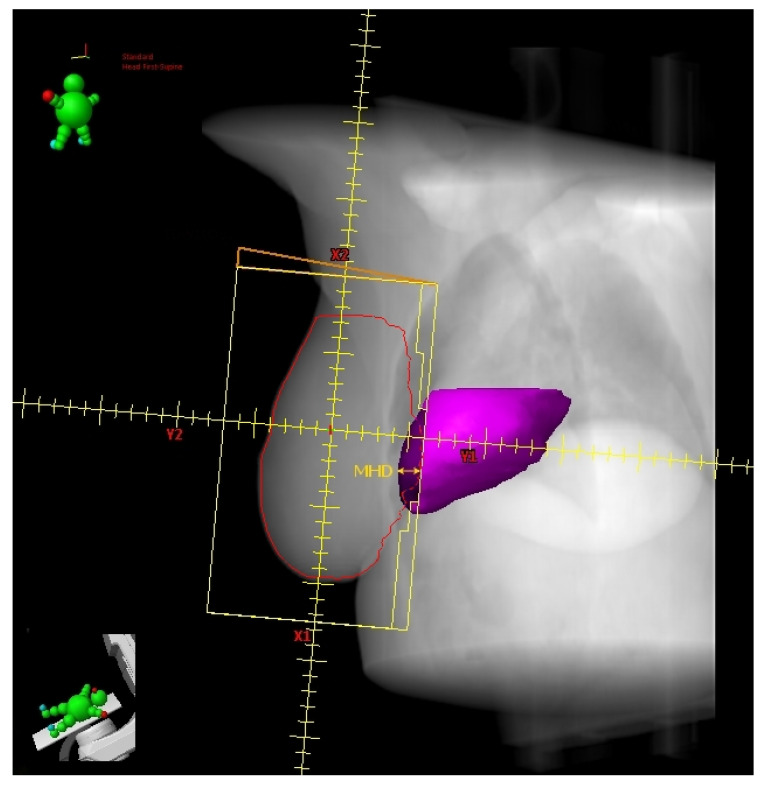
Digitally reconstructed radiograph (DRR) with external tangential beam’s-eye-view. The heart volume in field (HVIF, dark magenta) and the maximum heart depth (MHD) have been highlighted.
